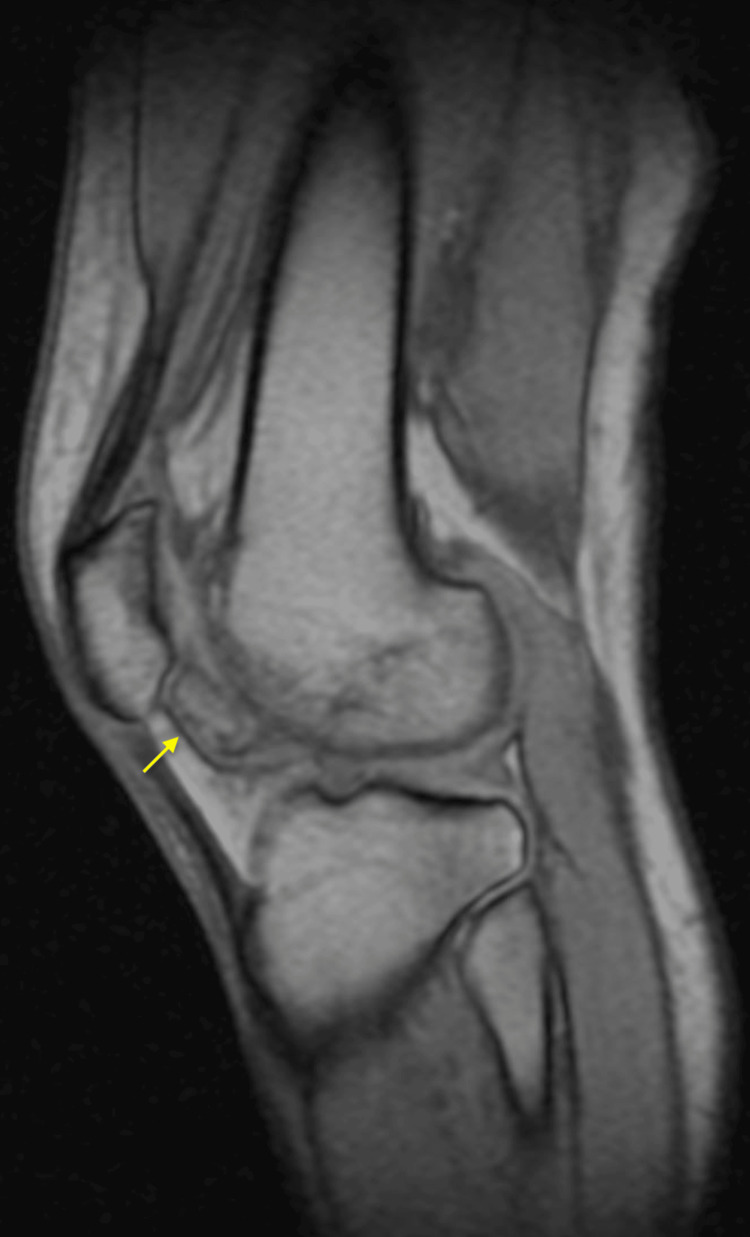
Magnetic resonance imaging of the left knee (sagittal view) showing an intra-articular loose body (yellow arrow).
